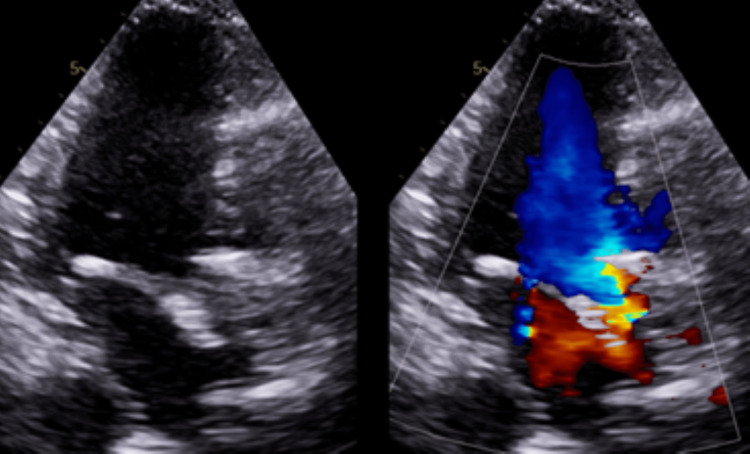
Eight Weeks Post TAVR. Thrombosed Valve while on Apixaban Ultrasound three-chamber long-axis view with color doppler showing flow acceleration across the bioprosthetic aortic valve. TAVR: transcatheter aortic valve replacement
